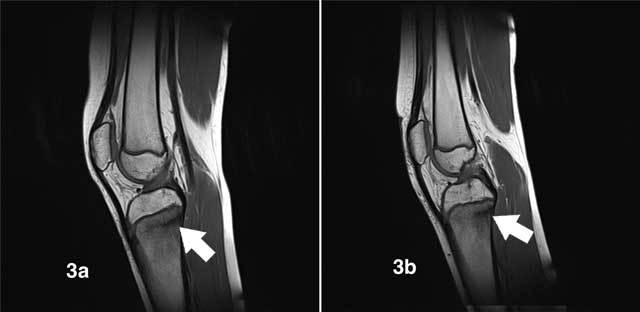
On T1-weighted images, the fracture lines are of low signal (white arrows) and are surrounded by bone marrow edema, which is of relatively low signal compared to normal bone marrow. a. Sagittal T1-WI of the right knee. b. Sagittal T1-WI of the left knee.
